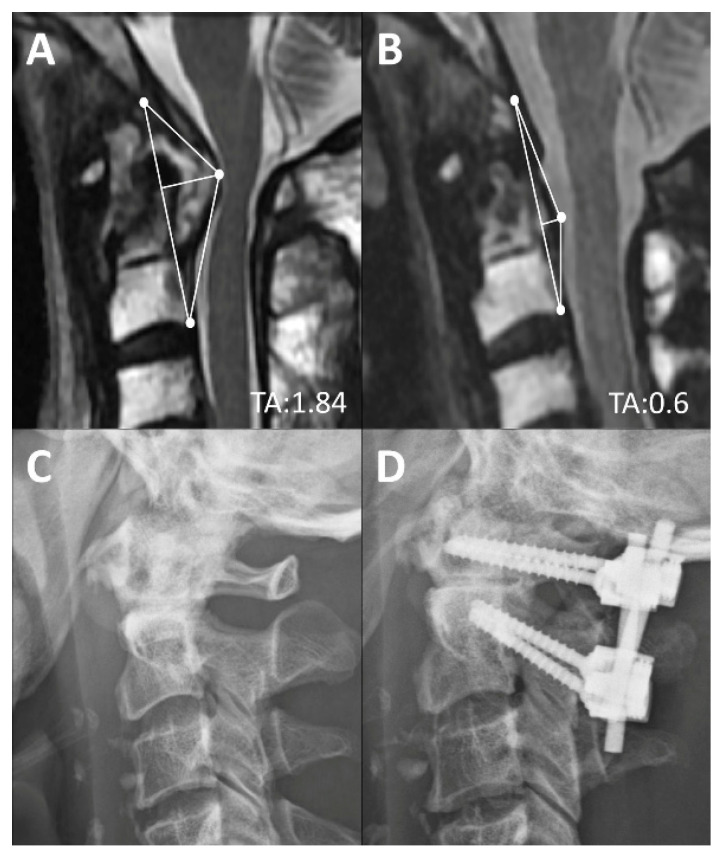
Pre-op (A) and 2-year post-op (B) mid-sagittal T2 magnetic resonance image of a rheumatoid arthritis patient with retro-odontoid pannus formation. Post-operatively, the base of the TA remained the same and the height decreased from 0.93 to 0.3 cm after regression of the pannus. Pre-op (C) and two-year post-op (D) lateral radiographs.
